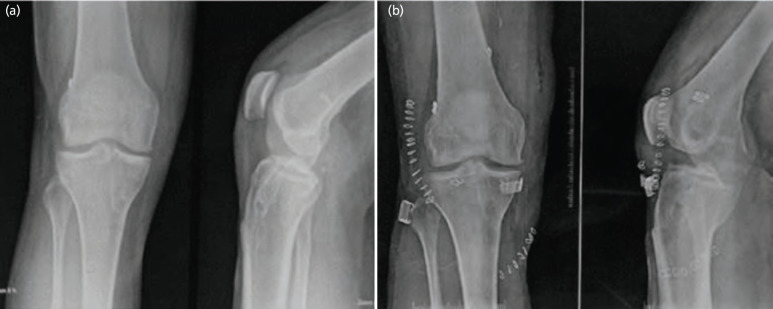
(a) Pre-operative radiograph of 26-year-old male, (b) post-operative radiograph of the same patient managed with revision ACLR + LET.
